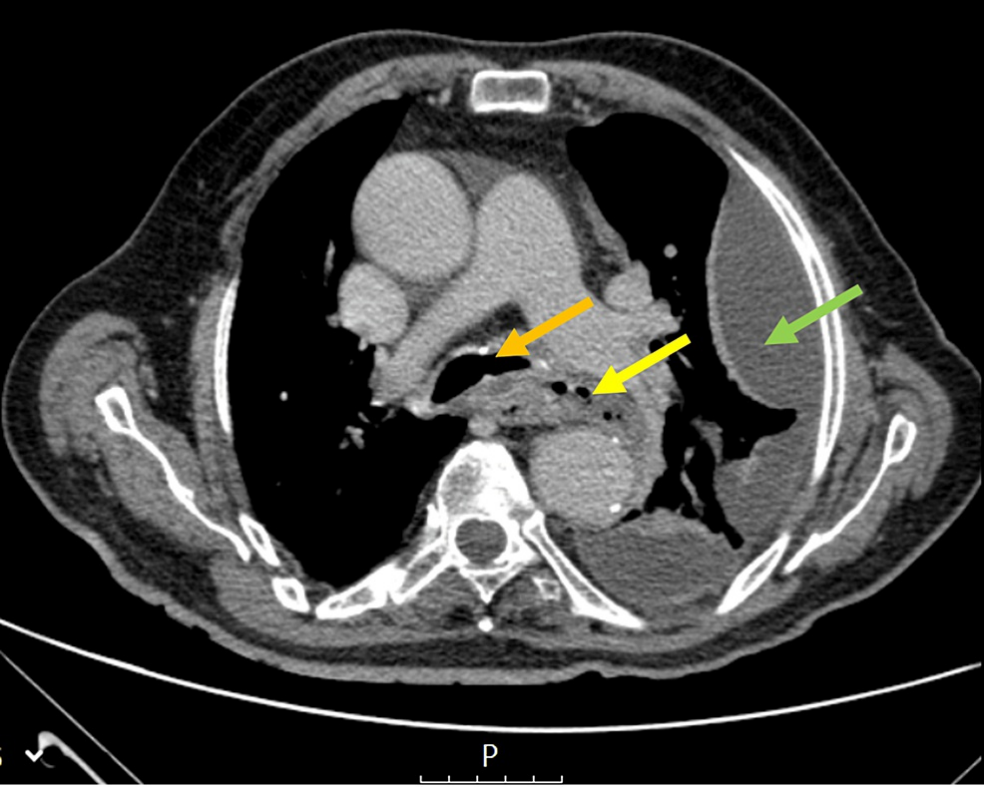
Computed tomographic angiography Yellow arrow: Infra-carinal esophageal rupture with pneumomediastinum, Green arrow: Left pleural empyema, Orange arrow: Carina
